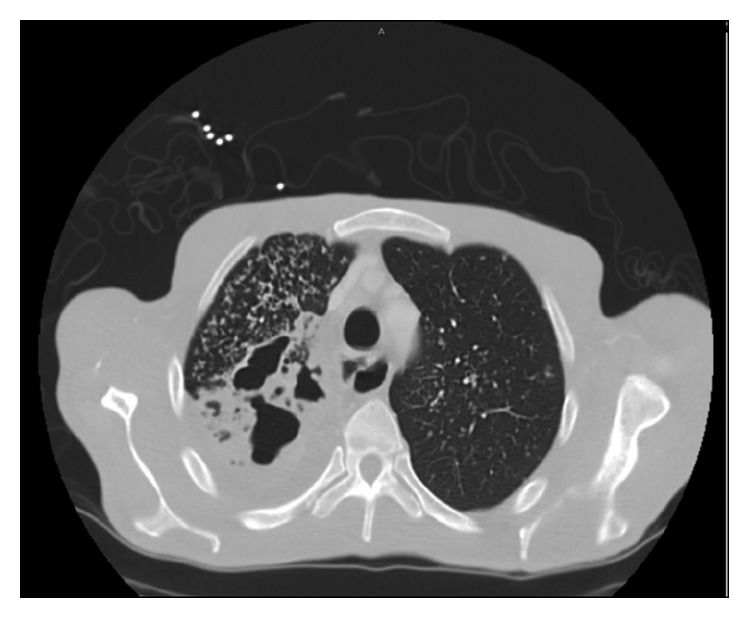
Contrast-enhanced CT scan. Axial section (neck) showing airspace consolidations with diffuse tree in bud opacities in the right lung apex and to a less extent, in the left lung apex. In addition, there are multiple areas of cavities within the right lung apex.
